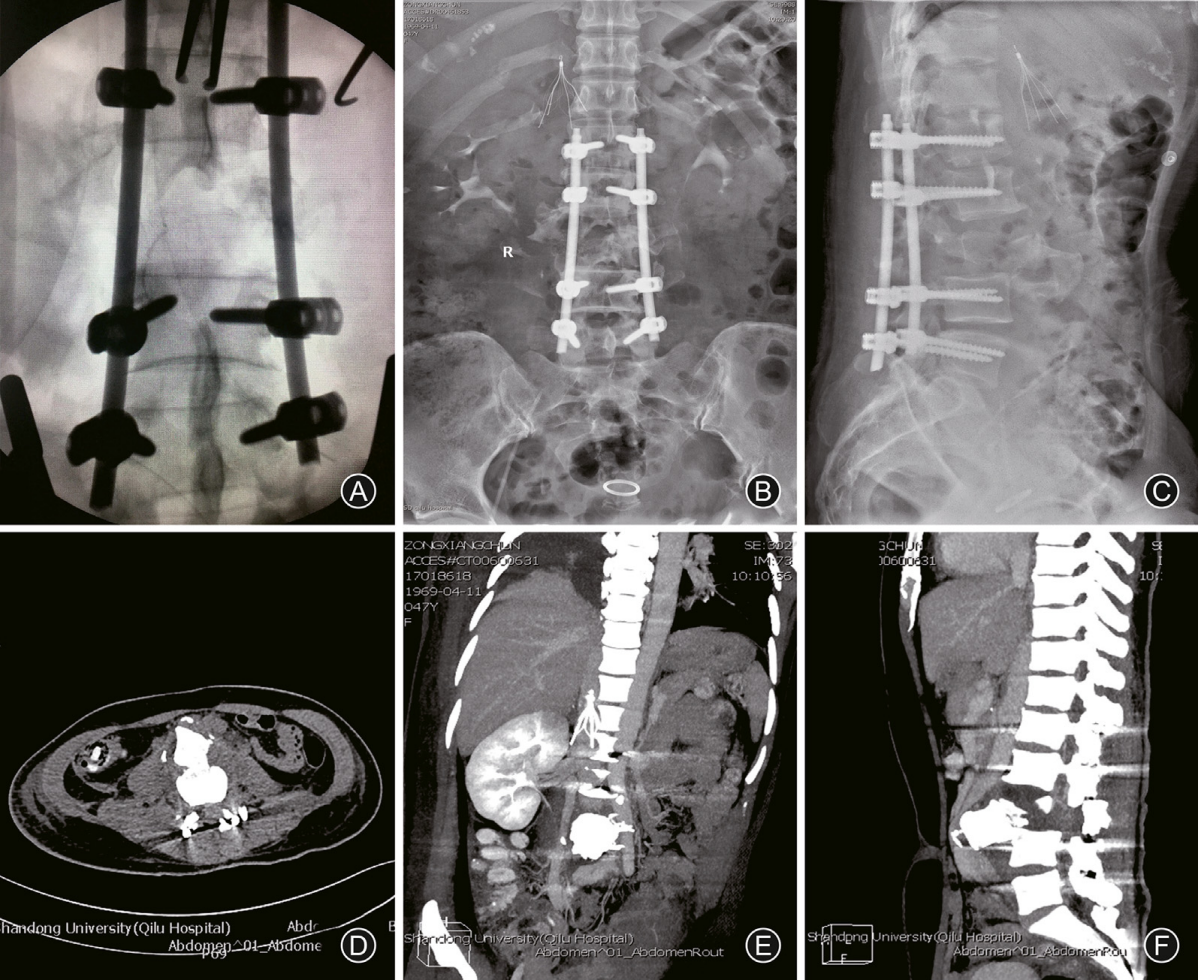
First posterior internal fixation. To stabilize the spinal alignment balance, posterior bilateral pedicle screw fixation and rods construction were performed on segment L_1_, L_2_, L_4_, and L_5_ vertebral bodies. Intraoperative fluoroscopy (A) and postoperative radiography, coronal (B) and sagittal (C), demonstrated successful restoration. Cut plane (D), coronal (E) and sagittal (F) CT scan revealed a mild retraction of L_3_ vertebra.
